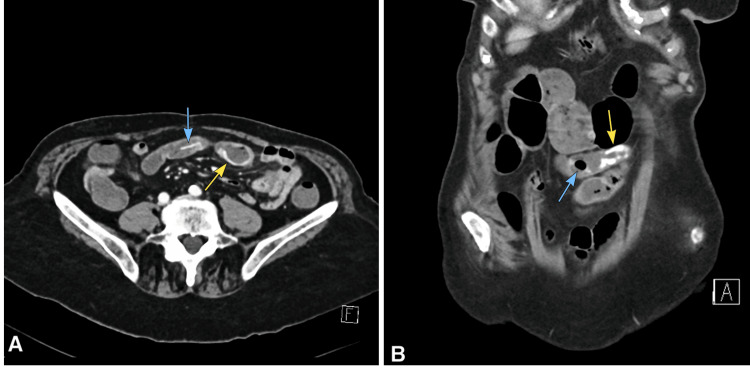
Portal venous phase contrast-enhanced abdominal CT scan at the time of the first follow-up Axial (A) and coronal (B) images showing the linear hyperdense structure (blue arrow) in the terminal ileum. Contrast extravasation is seen at the bowel wall, consistent with the source of bleeding (yellow arrow).
